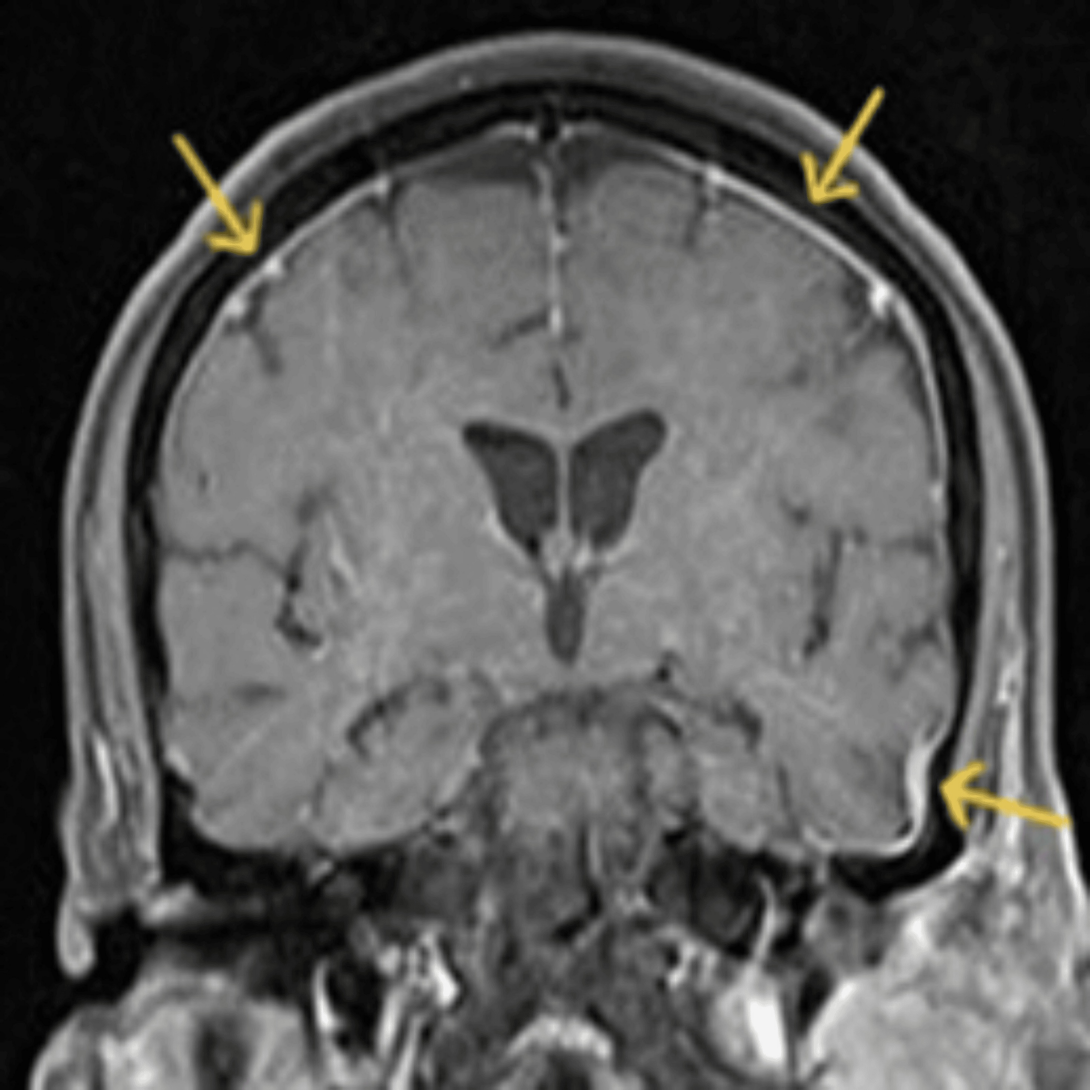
Coronal gadolinium-enhanced T1-weighted MRI Arrows depict the meningeal enhancement.
